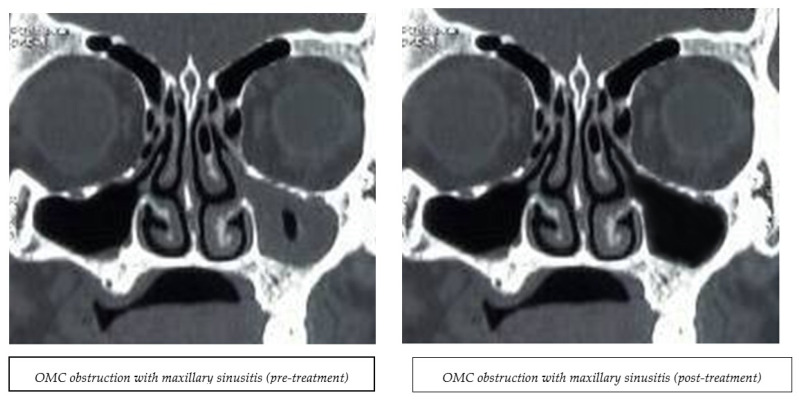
Pre- and post-CT findings after EMFT treatment.
